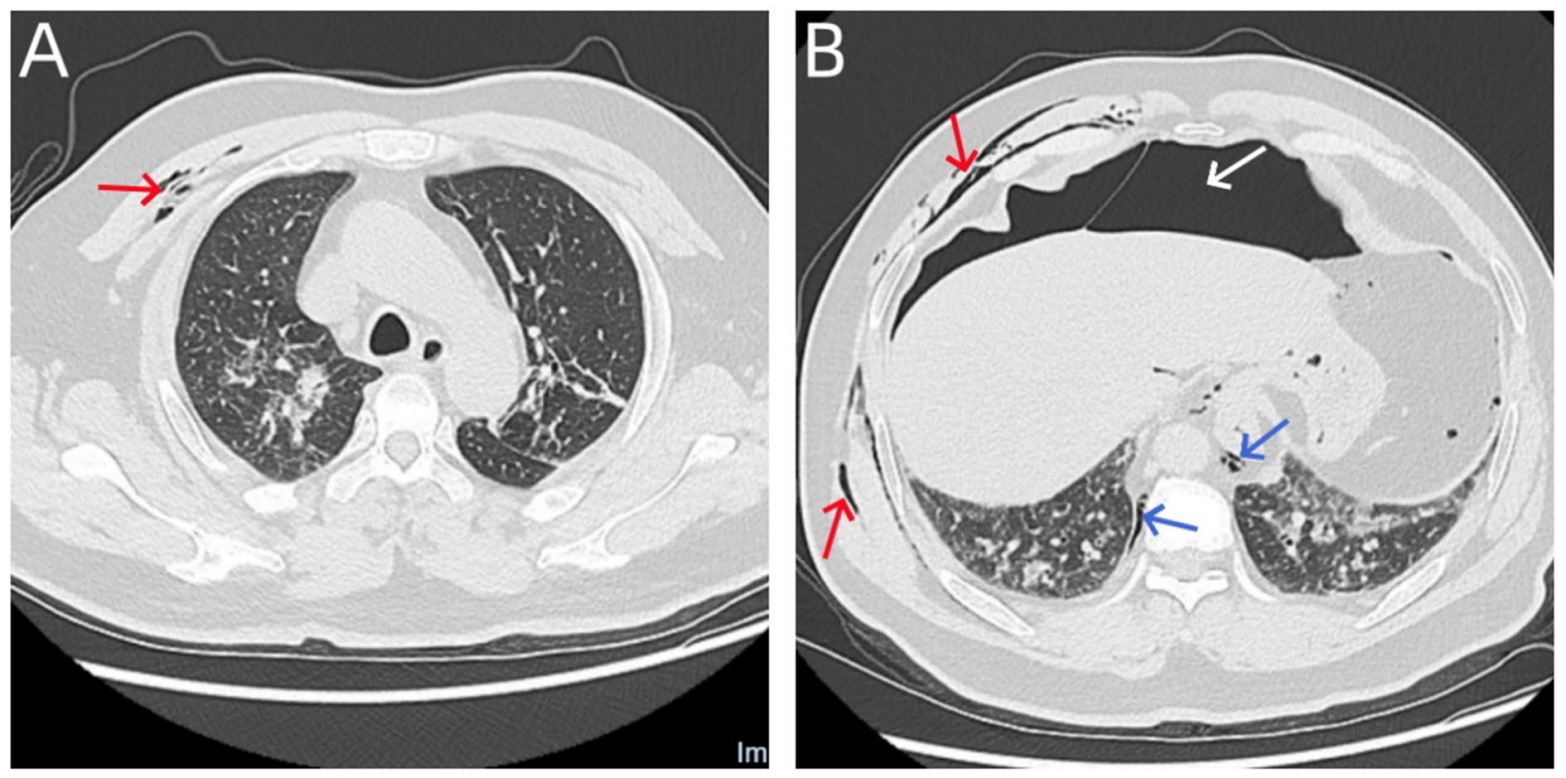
(A,B) Chest CT scan performed on the day of emergency treatment revealed multiple air collections in the right thoracoabdominal wall and muscle compartments (shown by red arrows), minimal air accumulation in the mediastinum (shown by blue arrows), and a large amount of free gas in the abdominal cavity (shown by white arrow). However, no signs of pneumothorax were observed.
